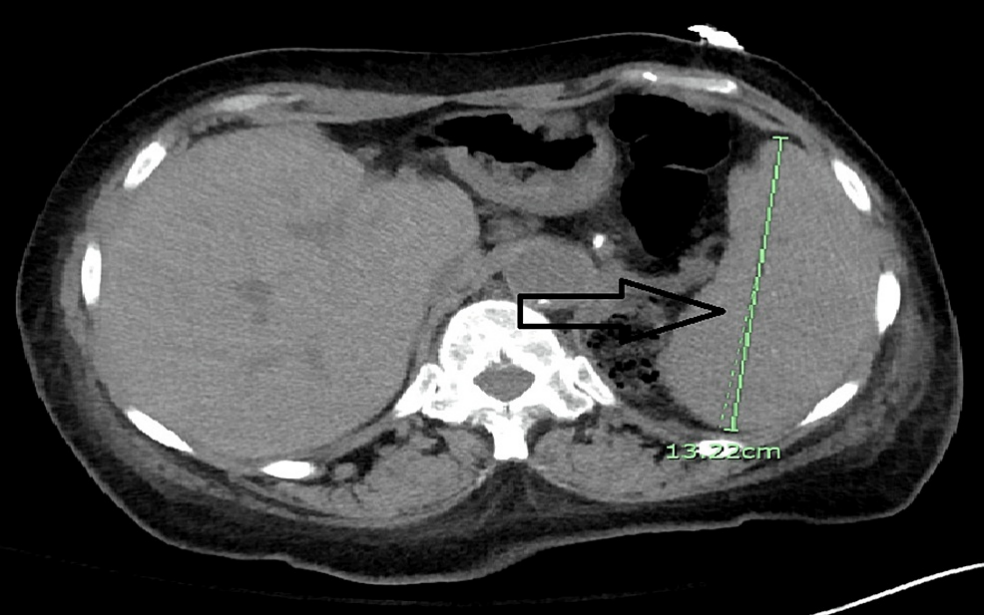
Computed tomography scan of the abdomen showing enlarged spleen.
